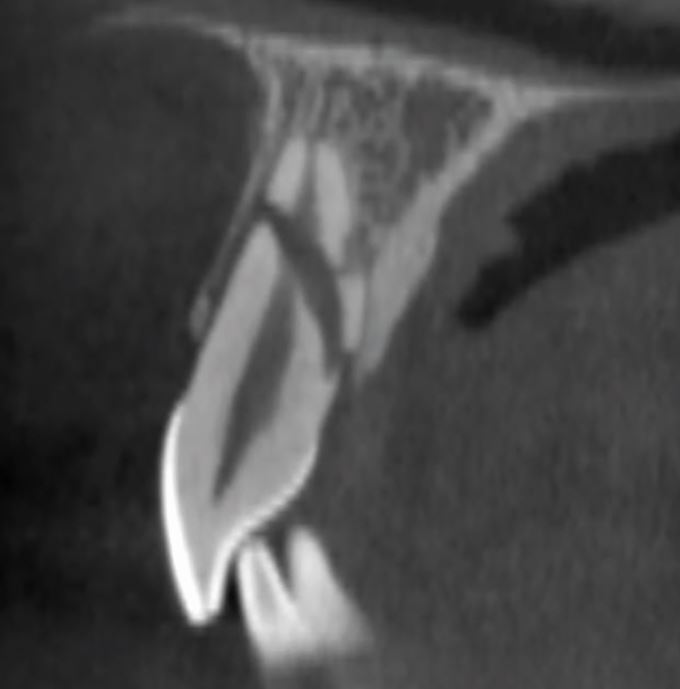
A CBCT scan of a root fracture, seen in the sagittal plane
